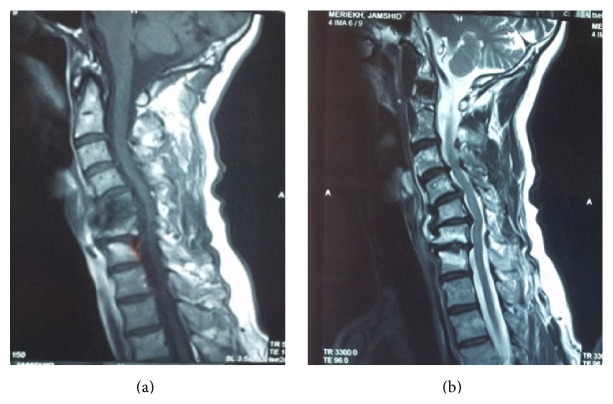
T1- and T2-weighted MRI and sagittal image in 2011 which had revealed cervical spondylosis with mild spinal cord compression.
